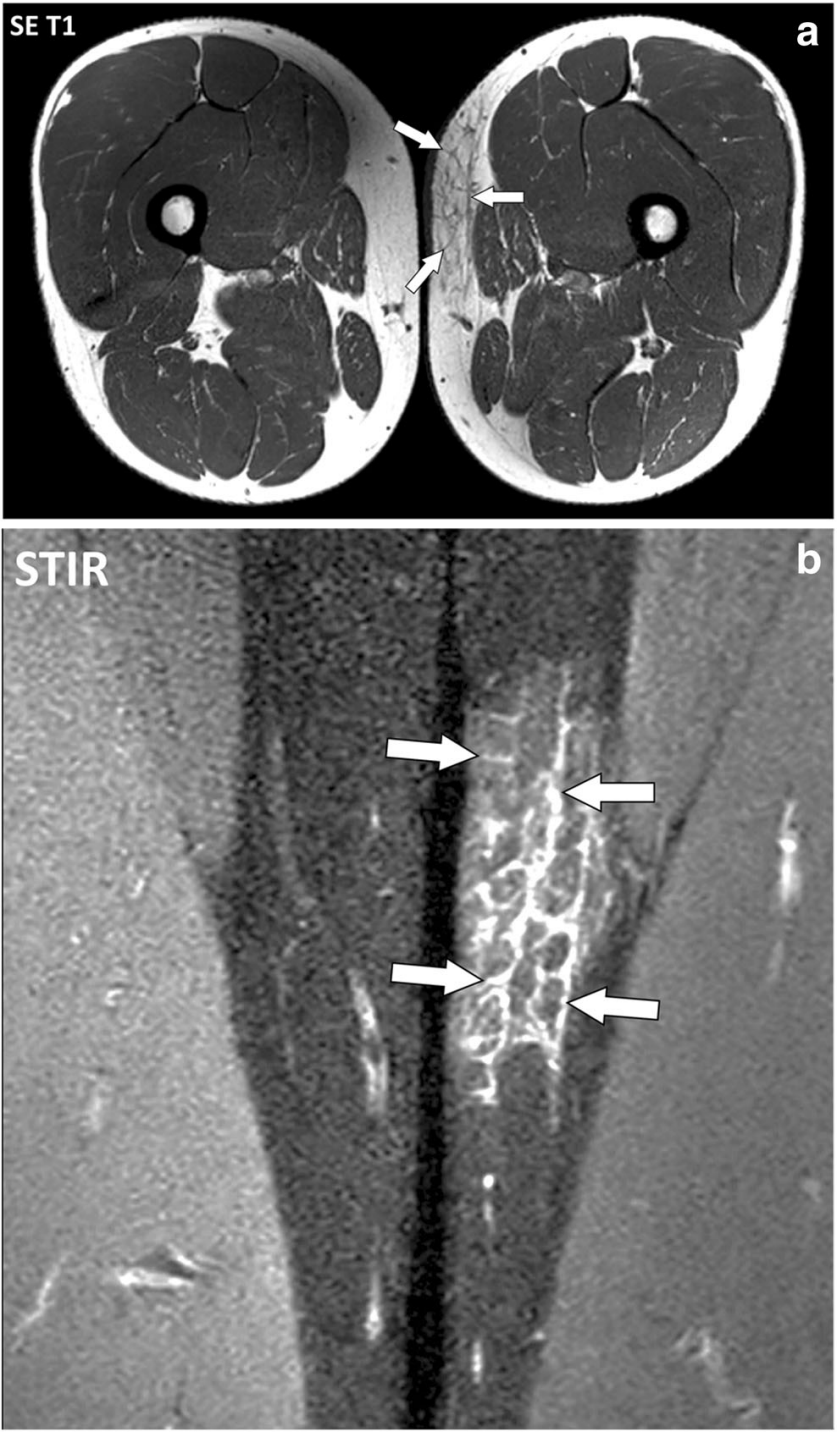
a Axial SE T1-weighted and (b) close-up coronal STIR images of the thighs of a 43-year-old male with dermatomyositis. The fascia superficialis of the left thigh (arrows) is thickened with fluid signal intensity
